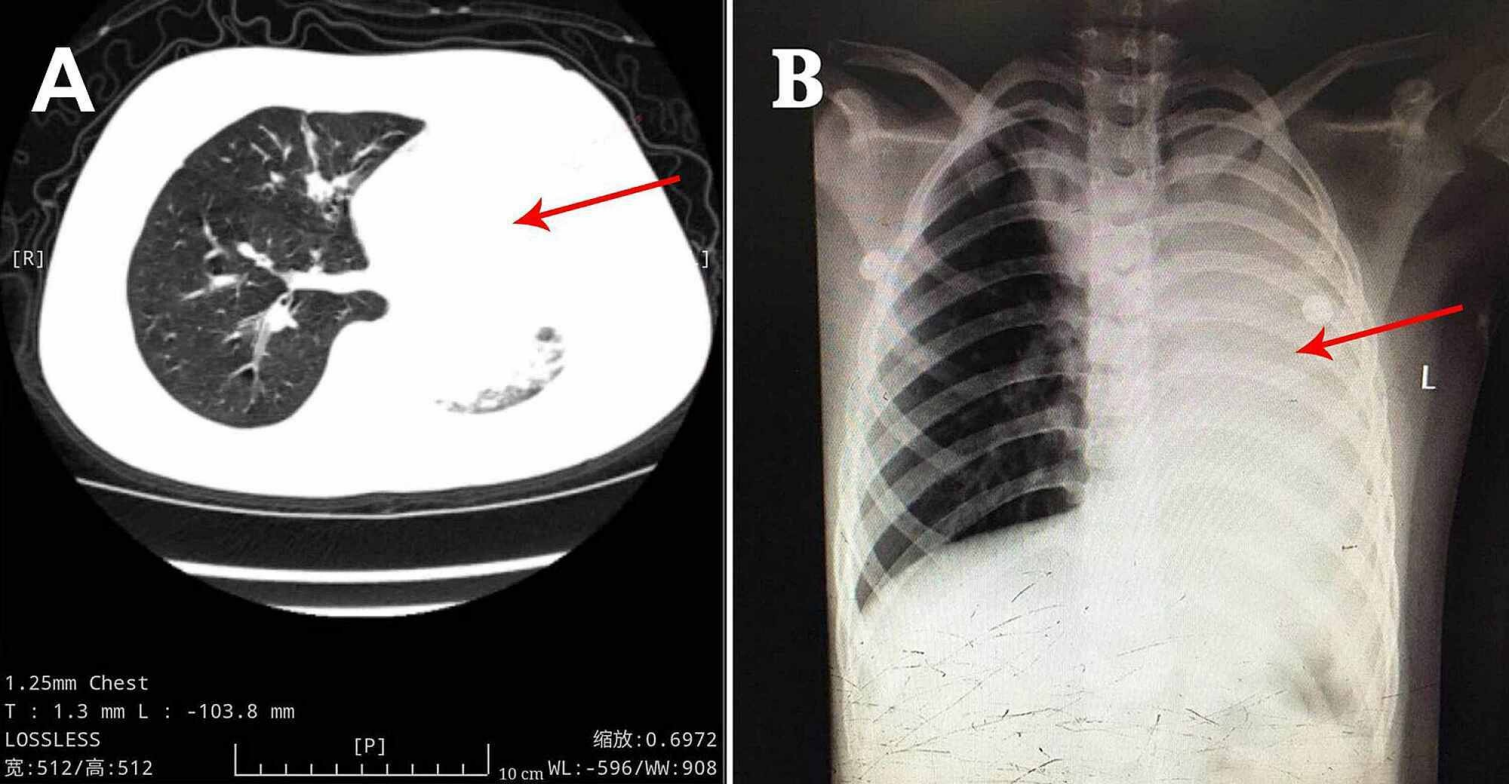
Primary Chest Computerized Tomography and Chest Radiograph (A) Chest CT at the local hospital showed atelectasis in the left lung and pneumonia in the right lung and (B) chest X-ray after mechanical ventilation revealed pneumonia in both lung lobes and atelectasis in the left lung.
